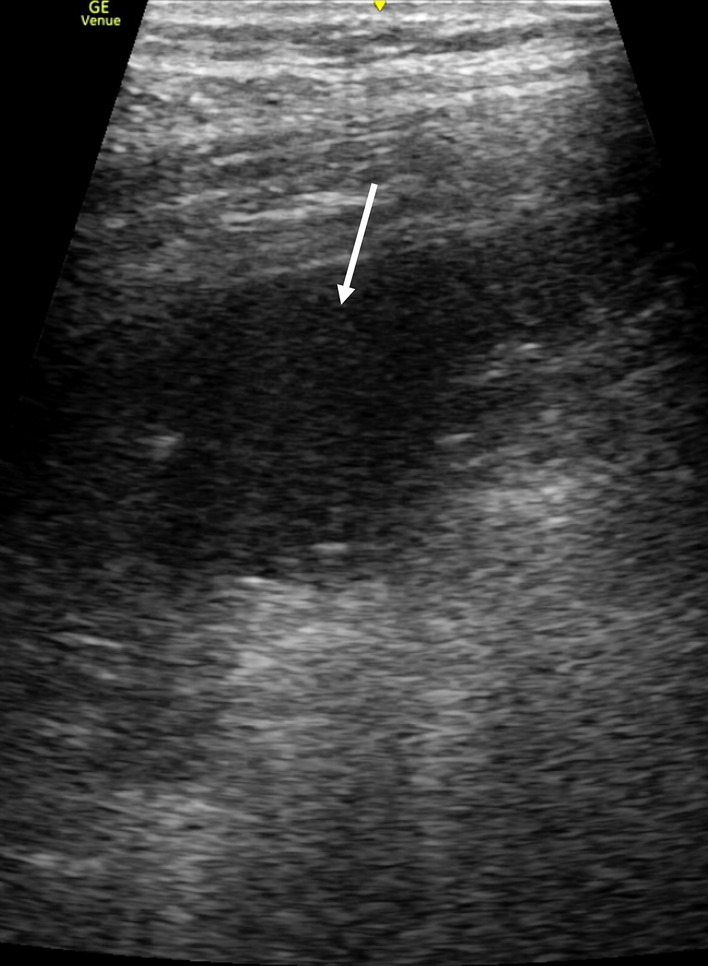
A lung ultrasound image of COVID-19 pneumonia showing sonographic consolidation in a gravitational region (arrow)
